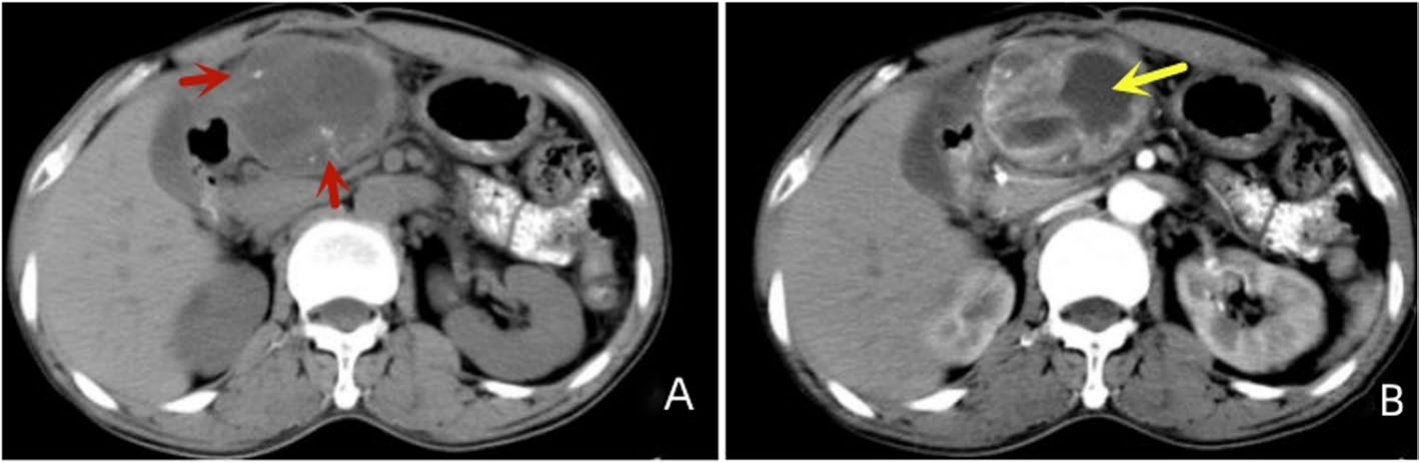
A 56-year-old man with EOS in the lesser omental bursa.  A, B CT showing a mass in the lesser omental cyst area, with coarse-grained calcification/ossification (red arrow) and cystic degeneration (yellow arrow); the mass was close relative to the gastric antral wall
